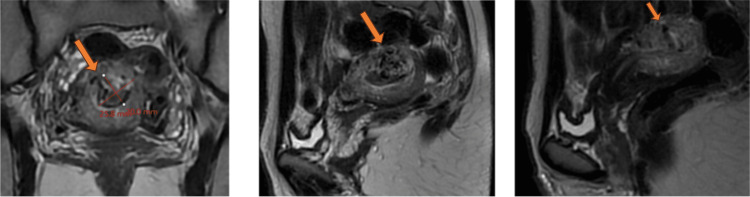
MRI of the pelvis with contrast showing the uterus is retroverted measuring 8.5 × 8 × 4 cm. Intrauterine intracavitary mass-like lesion measures 3 × 2.7 × 2.5 cm, collectively formed of multiple serpentine flow-related signal voids. It extends from the right parametrium through the anterior uterine wall mid-corporal to the endometrial cavity extending further to the left parametrium. A post-contrast examination shows the abnormal complex enhancement of serpentine vessels. Findings are suggestive of uterine arteriovenous malformation.
